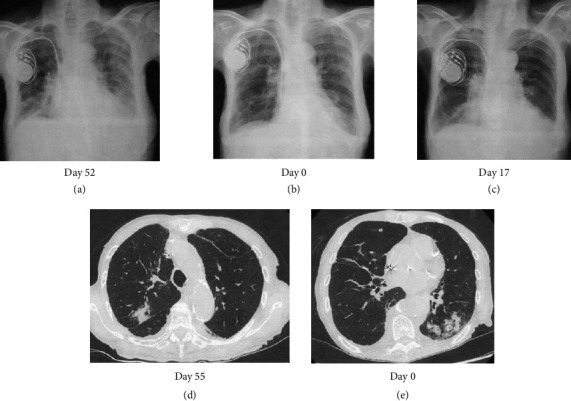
Chest X-ray before (a) and after (b) onset and before resurgence (c) of AFVD showed questionable lung infiltration. Chest CT before (d) and after (e) onset of AFVD clearly showed lung infiltration compatible with bacterial pneumonia. The number of days is calculated starting from the time of the patient's current hospitalization.
